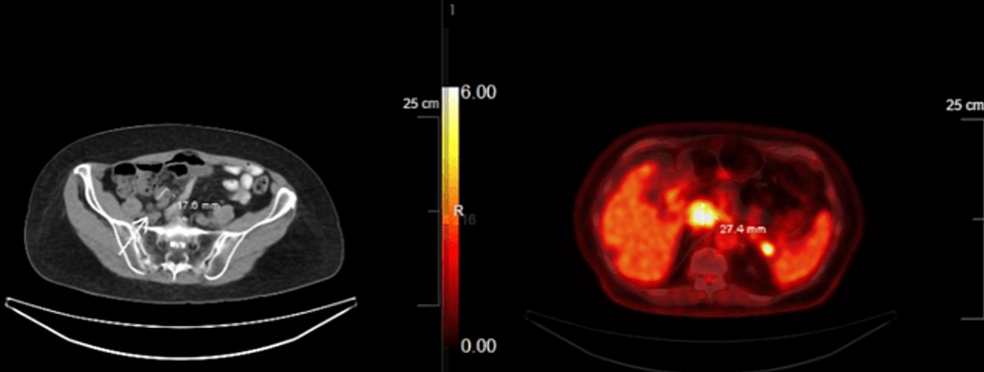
Additional CT sagittal views revealed a cecal mass with fluorodeoxyglucose-18 (FDG-18) uptake.
